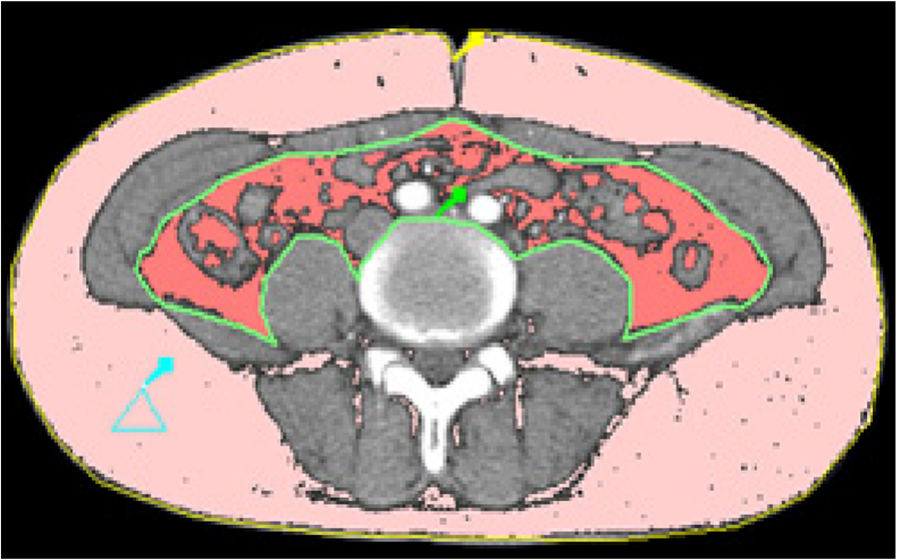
Distribution of abdominal fat as measured by FatScan software on a CT image. The visceral fat was indicated in red, and the subcutaneous fat was indicated in pink.
